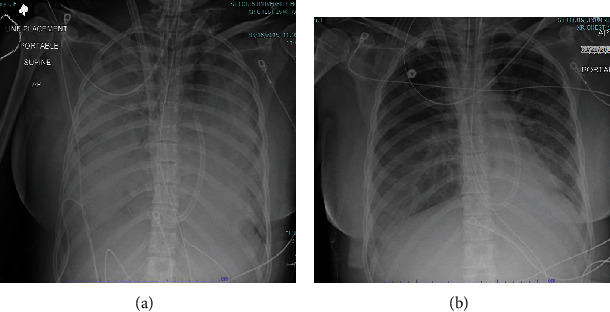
(a) Chest radiograph showed diffuse bilateral alveolar infiltrates on day 3 after the intoxication. (b) Chest radiograph showed significant improvement in bilateral infiltrates on day 5, right before the ECMO decannulation.
